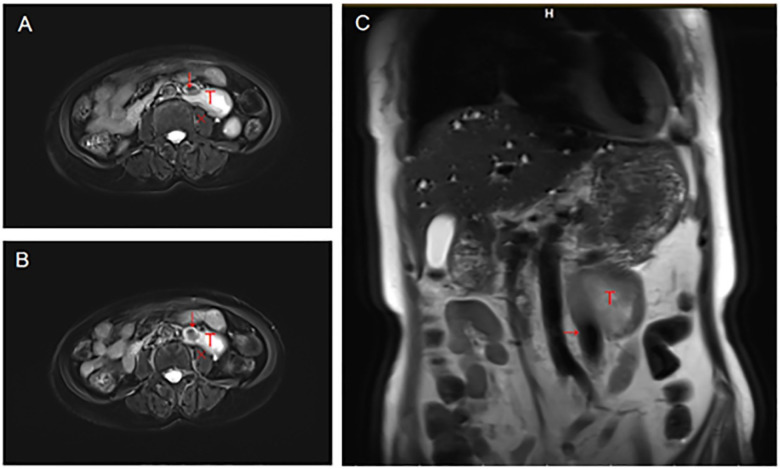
T2 MRI, (A) and (B) is transverse plane, (C) is coronal plane. Arrow indicates IVC, T indicates tumor, Fork indicates psoas muscle.
